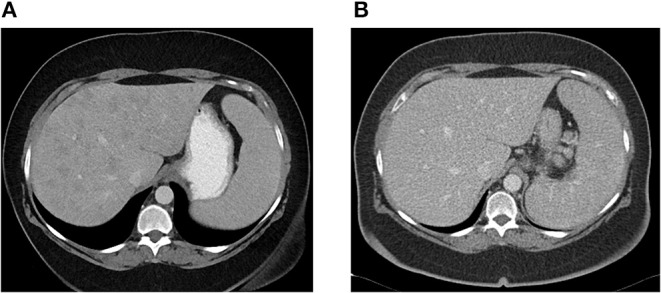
(A) Abdominal CT showing innumerable low attenuation nodules consistent with hepatic sarcoidosis. Patient was previously diagnosed with AMA-negative PBC, later re-evaluated and diagnosed as sarcoidosis. (B) Abdominal CT 6 months later, after patient was successfully treated with azathioprine with resolution of hepatic nodules.
